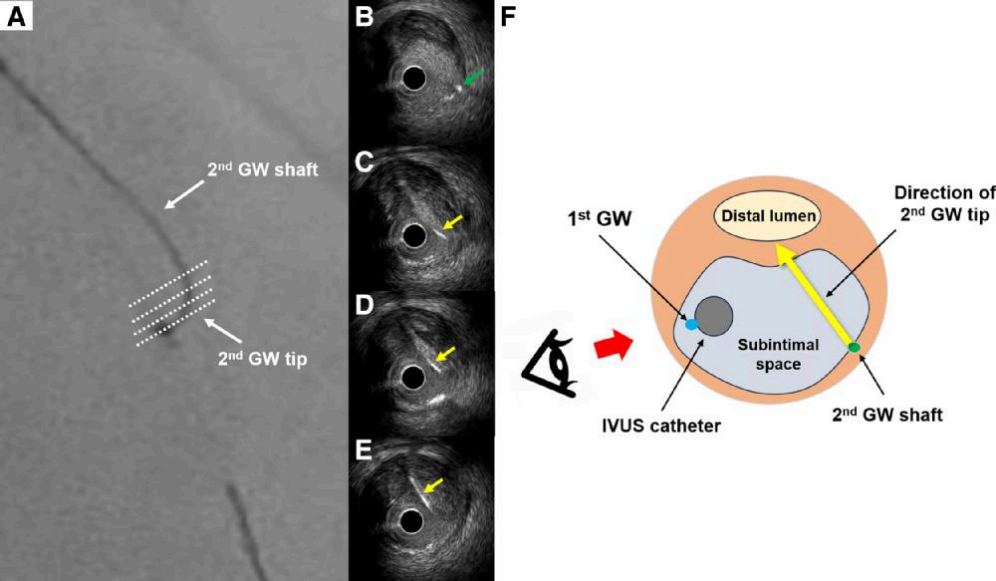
Confirming the fluoroscopic viewing direction based on the positional relationship between the IVUS catheter and second guidewire. (*A*) The second guidewire shaft is located on the right side of the intravascular ultrasound (IVUS) catheter, and its tip is towards the IVUS transducer. (*B–E*) Serial IVUS cross-sectional images from proximal to distal left descending coronary artery. (*F*) The fluoroscopic viewing direction on IVUS images is from 8 o’clock.
